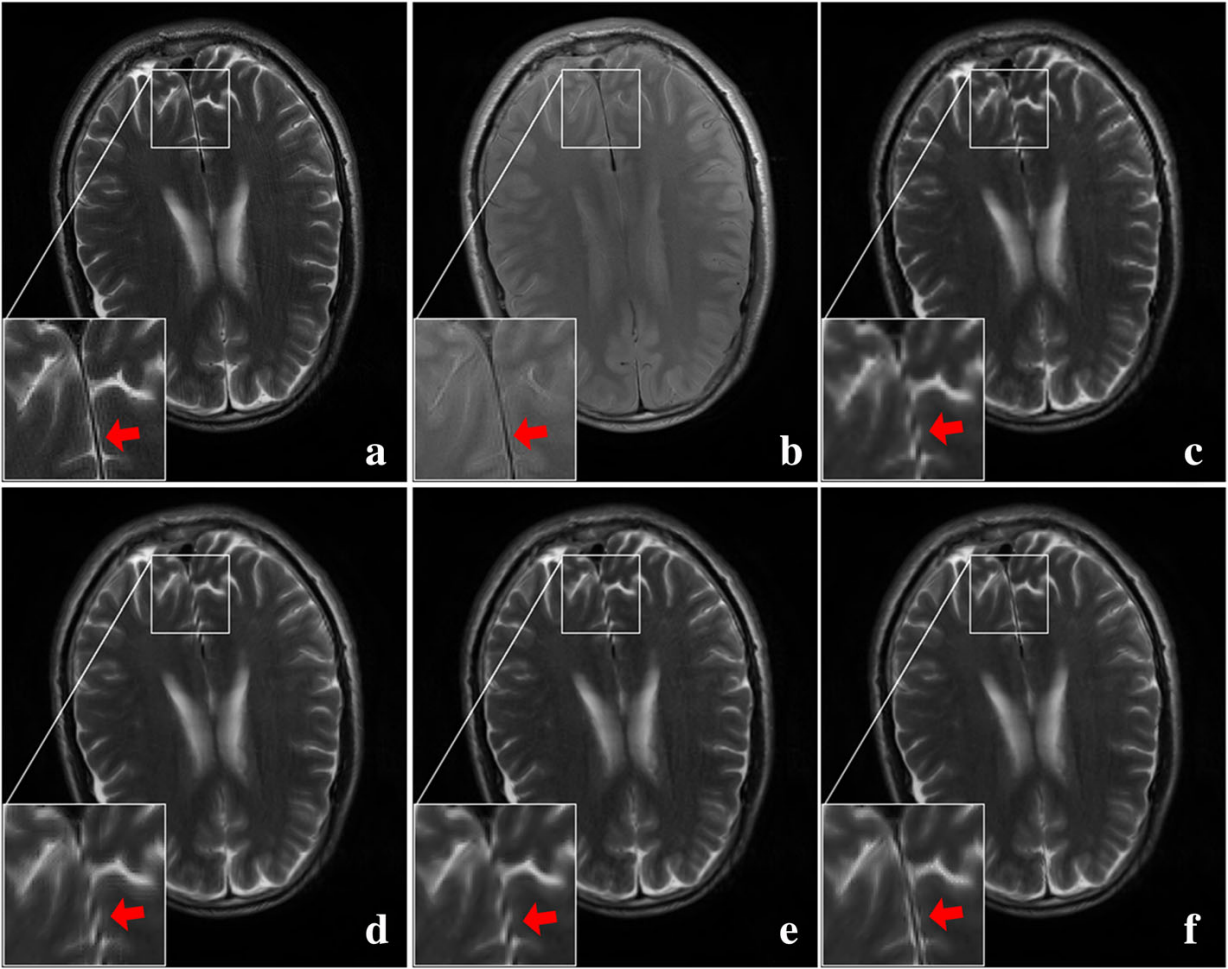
One pair of T1 and T2 MRI images acquired on 3 T Siemens scanner. a HR of T2 image; b HR of T1 image; c-f are super-resolved images using the bicubic, NEDI, CGI, and the proposed method, respectively
